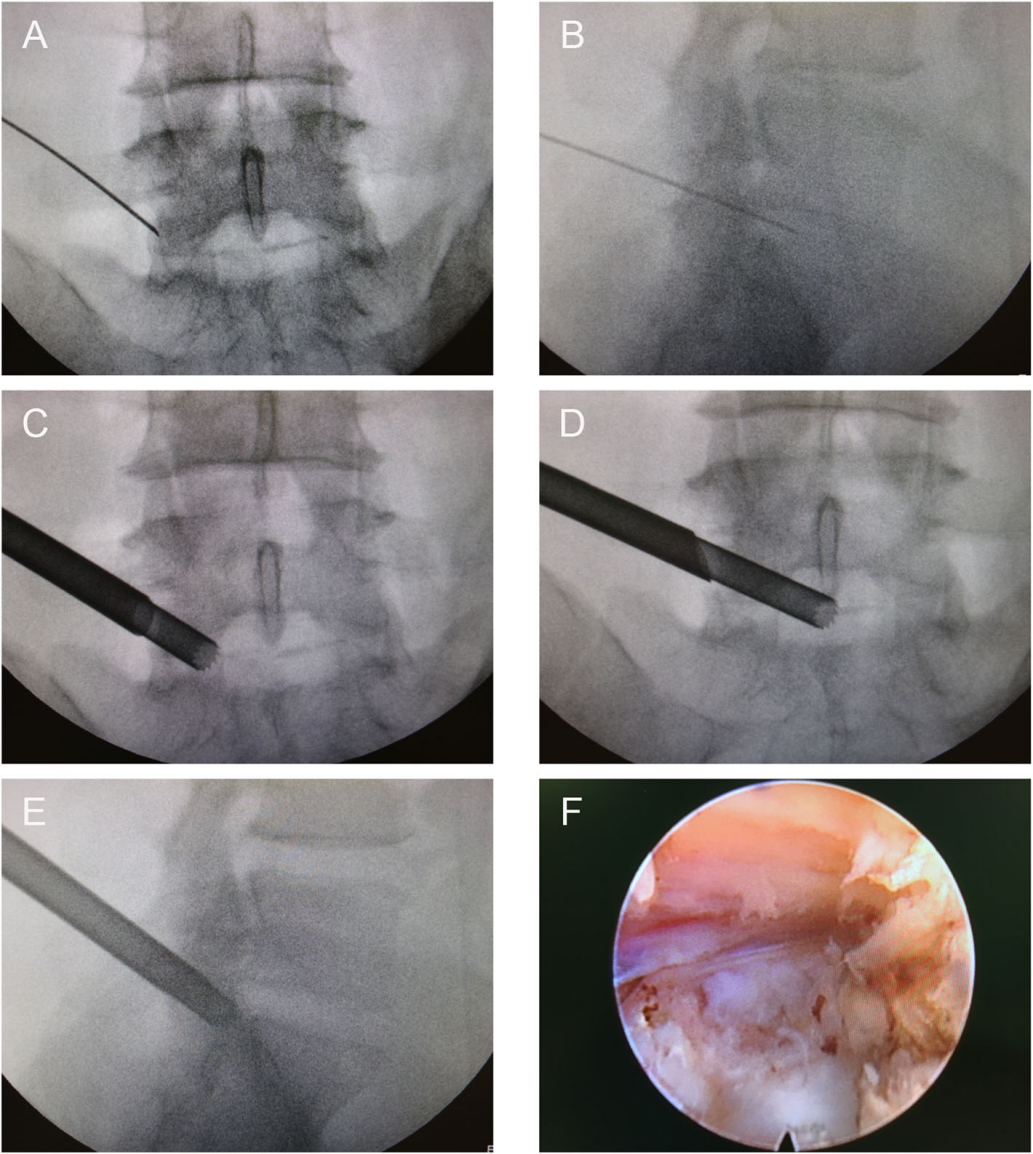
Intraoperative X-ray and endoscopic images of the surgery. a Posteroanterior X-ray image of the puncture procedure. b Lateral X-ray image of the puncture procedure. c Drill the trephine until the distal end reaches the medial border of the pedicle on posteroanterior view. d, e Press down the trephine further and keep drilling until the distal end exceeds the midpoint between medial border of the pedicle and the spinous process on posteroanterior (d) and lateral (e) view. f Endoscopic view after removing the herniation
